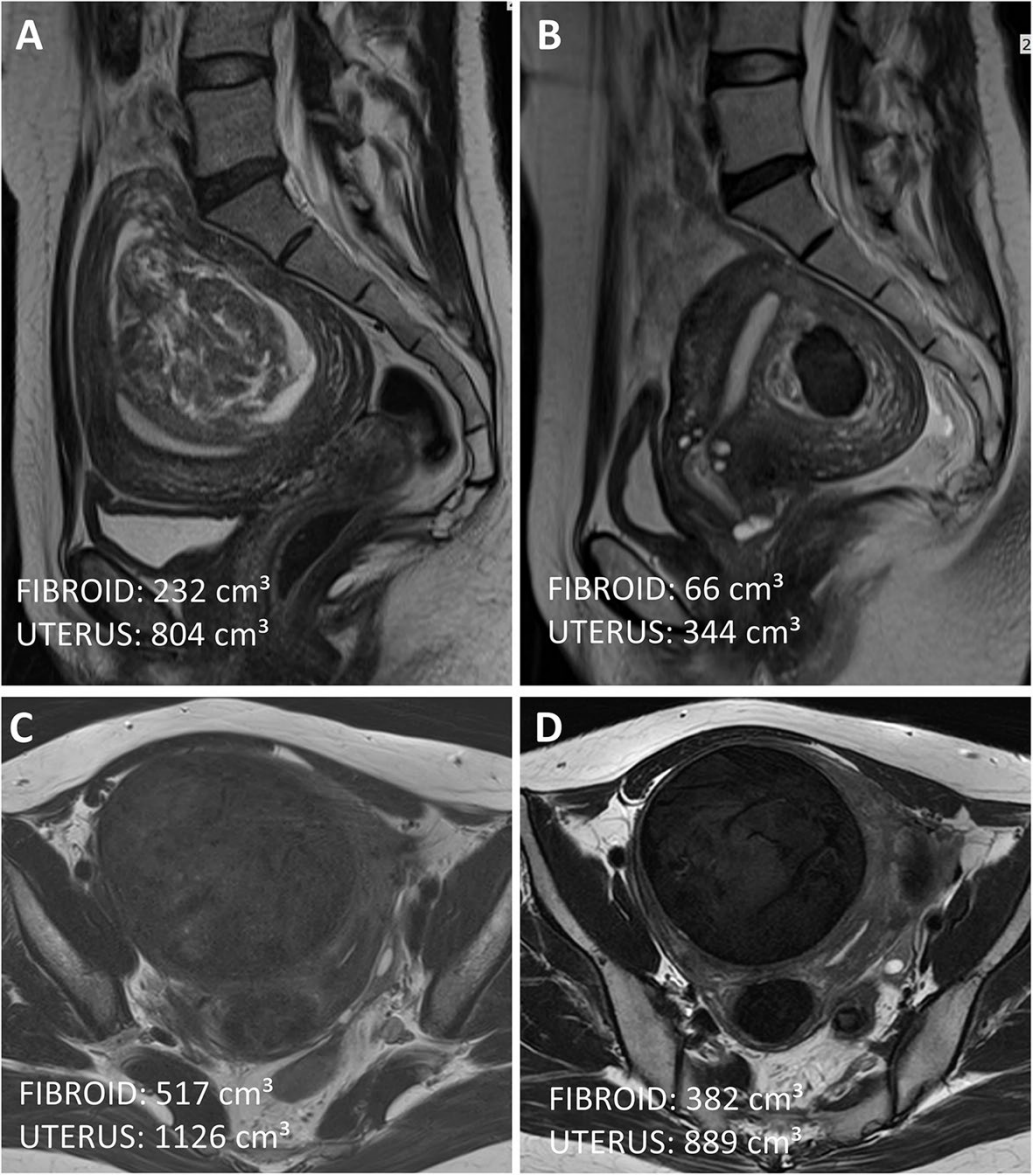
MRI assessment of the uterine and fibroid volume post-UAE. FIGO 3 fibroid showed a 71% volume, and uterine volume decreased by 57% from baseline (A) to 6 months after UAE (B). FIGO 4 fibroid had a 26% volume reduction, with a 21% decrease in uterine volume from baseline (C) to 6 months after UAE (D). Menstrual symptoms improved notably after UAE; the patient with FIGO 3 fibroid experienced a reduction in menstrual flow (from 6/10 to 3/10) and pain (from 2/10 to 0/10). The patient with FIGO 4 fibroid reported a decrease in menstrual flow (from 10/10 to 7/10) and pain (from 10/10 to 5/10) at the 6-month follow-up
